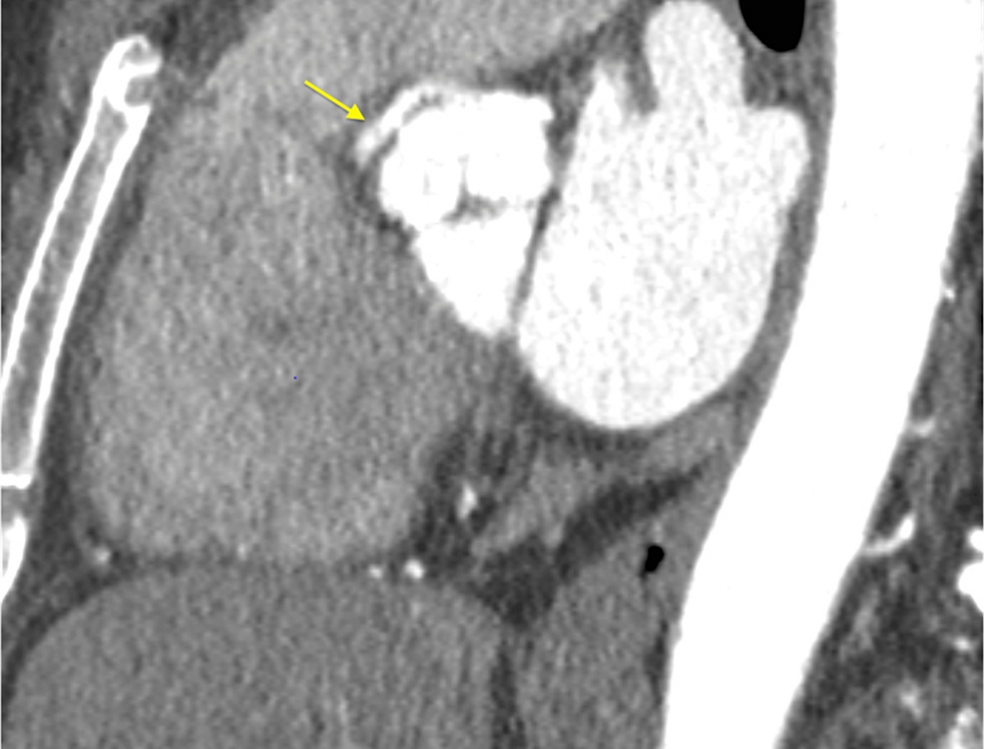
Cardiac computed tomography angiography (CCTA) demonstrating an anomalous RCA originating from above the left sinus of Valsalva with an interarterial course between the aorta and pulmonary artery. The yellow arrow indicates the anomalous RCA with an acute angle takeoff from the aorta. RCA: right coronary artery
